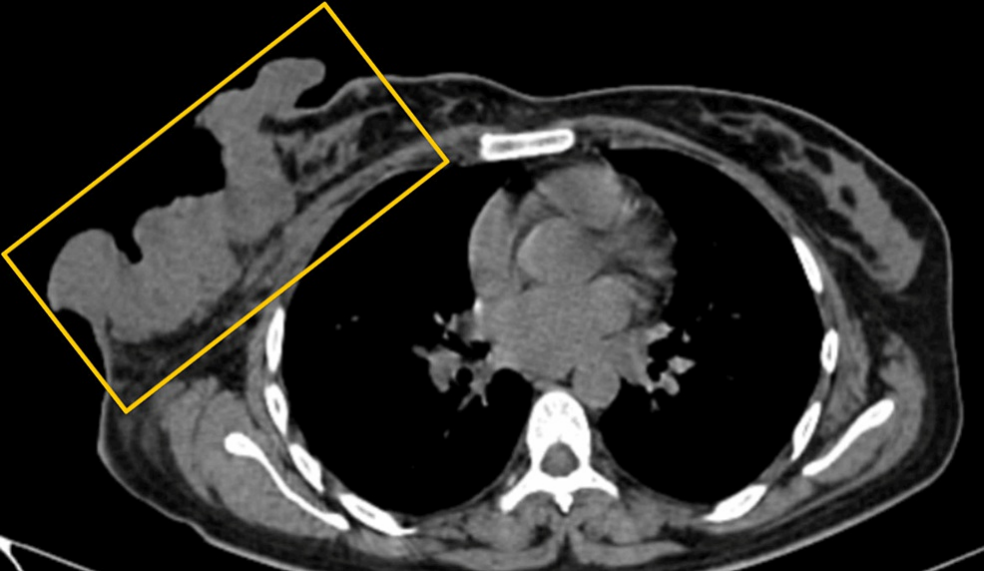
Computed tomography scan showing fungating growth invading the chest wall
